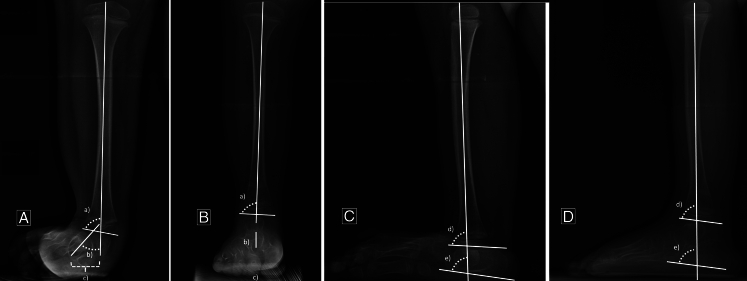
Radiologic measurement one of the patients before and 1 year after SUPERankle procedure. A, Radiologic measurement on AP radiograph in coronal plane before surgery—(a) mLDTA: 80 degrees; (b) mTCA: 40 degrees; (c) mTCD: 17 mm. B, Radiologic measurement on AP radiograph in coronal plane 1 year after surgery—(a) mLDTA: 89 degrees; (b) mTCA: 2 degrees; (c) mTCD: 0 mm. C, Radiologic measurement on lateral radiograph in sagittal plane before surgery—(a) mADTA: 85 degrees; (b) mLTCA: 80 degree. D, Radiologic measurement on lateral radiograph in sagittal plane 1 year after surgery—(a) mADTA: 82 degrees; (b) mLTCA: 82 degrees.
